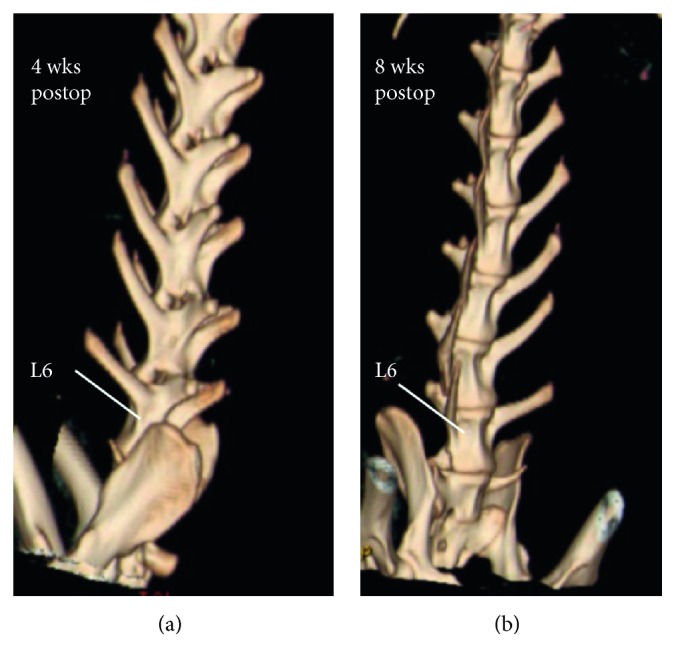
Representative 3D reconstructed CT images indicating no bony destruction of the vertebral body and calcification of the paravertebral soft tissue at 4 and 8 weeks postop, respectively.
